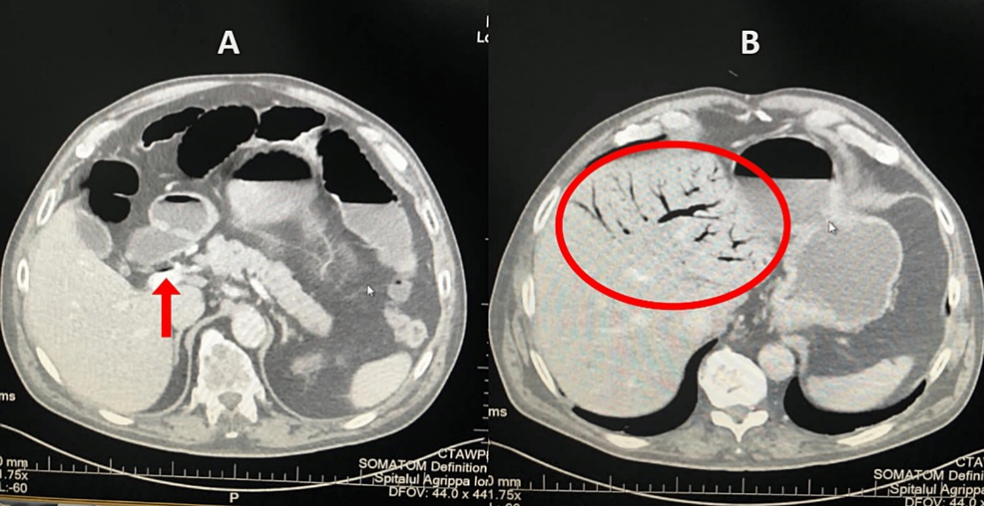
Abdominal/pelvic CT scan with contrast. (A) The arrow points to gas located inside the portal vein. (B) Cranially, gas can be seen inside the branches of the portal vein and inside the liver (circled part).
